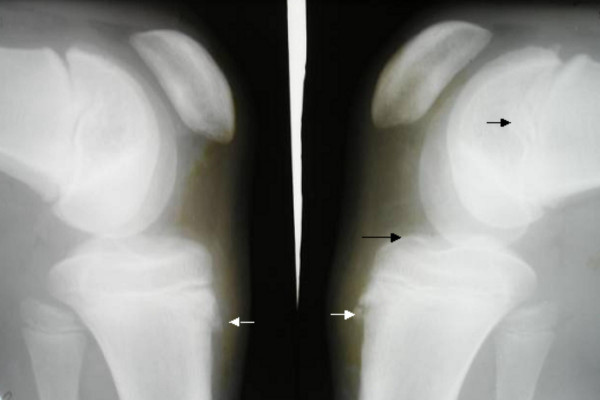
Patient 2: Lateral radiograph of the knee with the leg internally rotated (10–20°) showed bilateral irregularity of the apophysis with separation from the tibial tuberosity.
